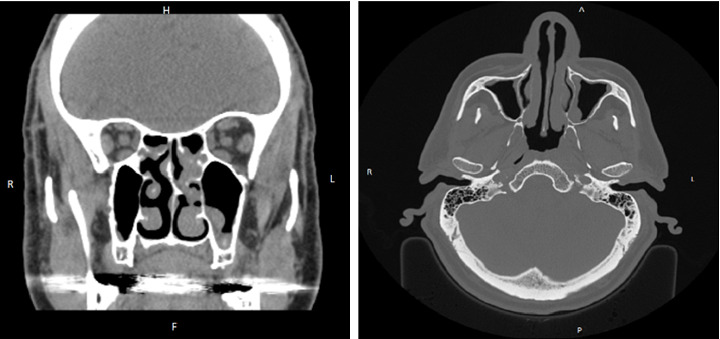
CT sinus study showed moderate to severe paranasal sinus disease, worst at the frontoethmoidal recesses and anterior ethmoid air cells. Leftward nasal septal deviation with bony spurring that contacts the left middle turbinate.
